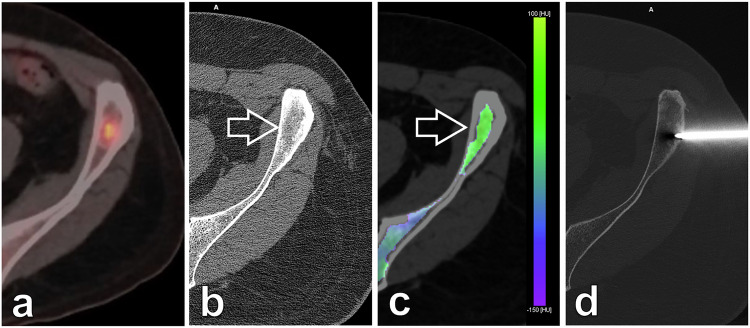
A 47-year-old male patient with a history of locally advanced adenoid cystic carcinoma showed an abnormal uptake focus in the left iliac blade on a positron emission tomography/CT follow-up scan (a) for which a bone biopsy was requested. b Monoenergetic axial 70-keV bone algorithm reconstruction (0.6-mm thickness; Br80) PCCT-guided biopsy image demonstrated a subtle sclerotic area (arrow) in the left iliac blade with no apparent identifiable bone lesion. c Real-time BME mapping (2-mm thickness; Qr40) depicted increased and pathological values, colour-coded in shades of yellow/green (arrow), while healthy marrow values are colour-coded in blue/violet as seen in the remote bone. In cases where a lesion detected by other imaging techniques does not translate on CT, real-time BME mapping allows for a confident intraprocedural bone biopsy guidance (d) towards the pathologic region of interest, documenting that the obtained samples followed the desired trajectory. Final diagnosis was metastatic disease. BME, Bone marrow oedema; PCCT, Photon-counting computed tomography
